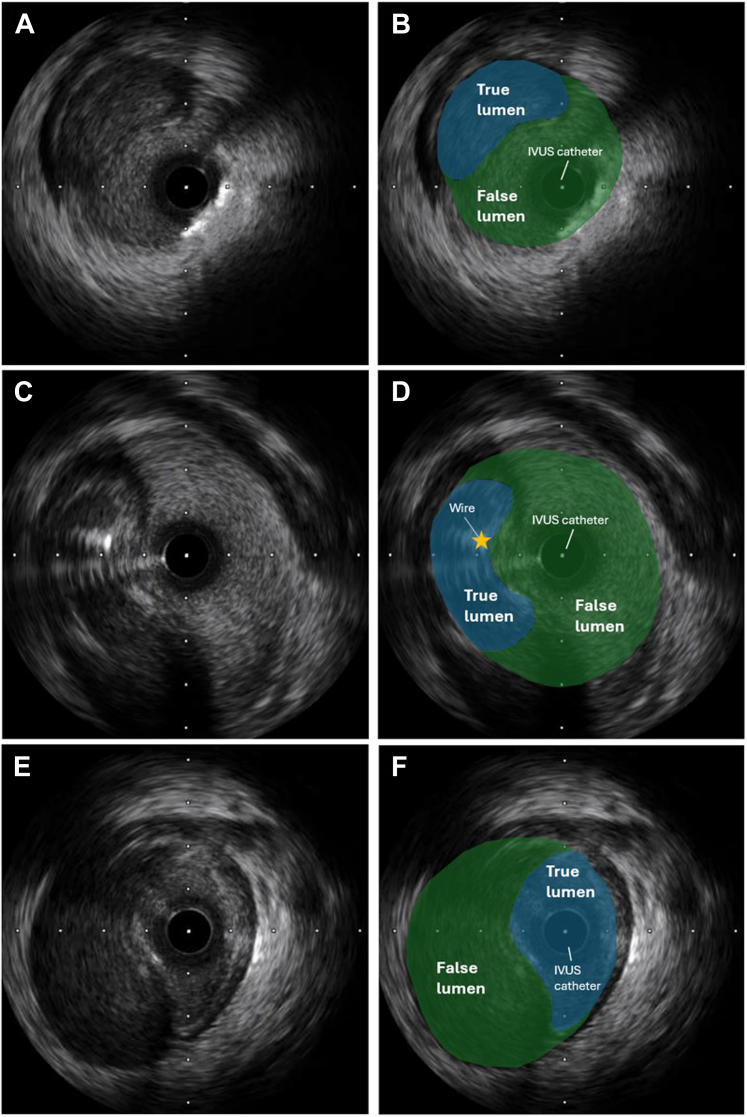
Intravascular Ultrasound Imaging demonstrating the intravascular ultrasound catheter (A and B) within the false lumen and (C and D) wiring of the true lumen. Confirmatory intravascular ultrasound was then performed (E and F).
